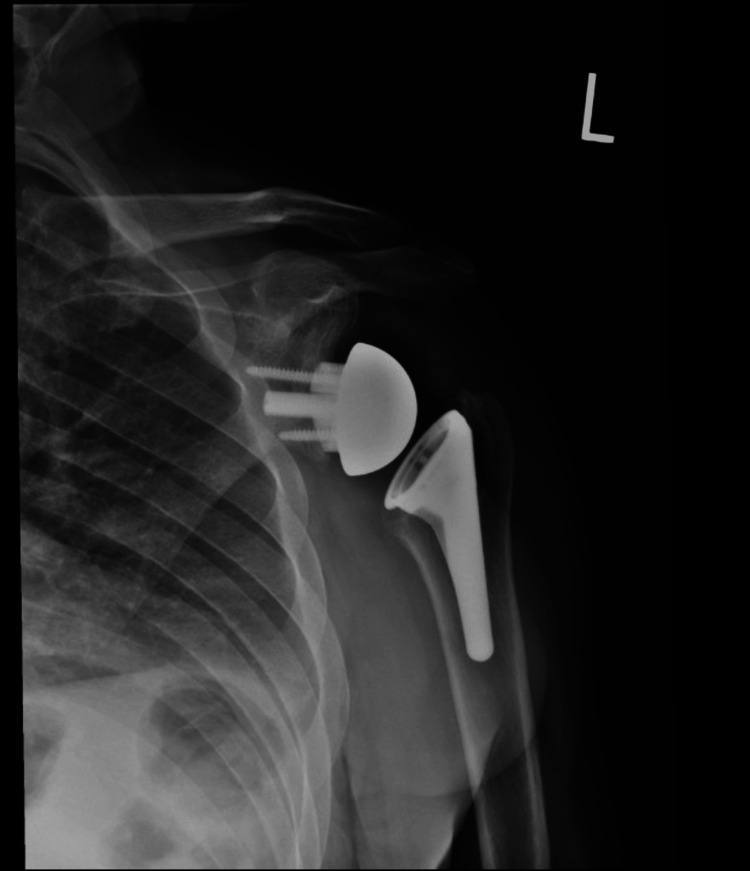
Postoperative X-rays of the right shoulder at one-year follow-up AP: anteroposterior The image depicts an AP view of the right shoulder
